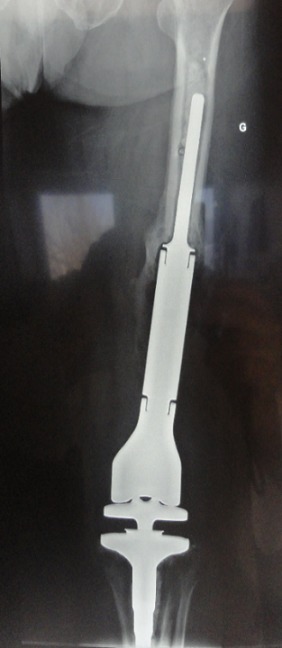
Anteroposterior radiograph showing good osseointegration of the megaprosthesis one year after knee replacement
